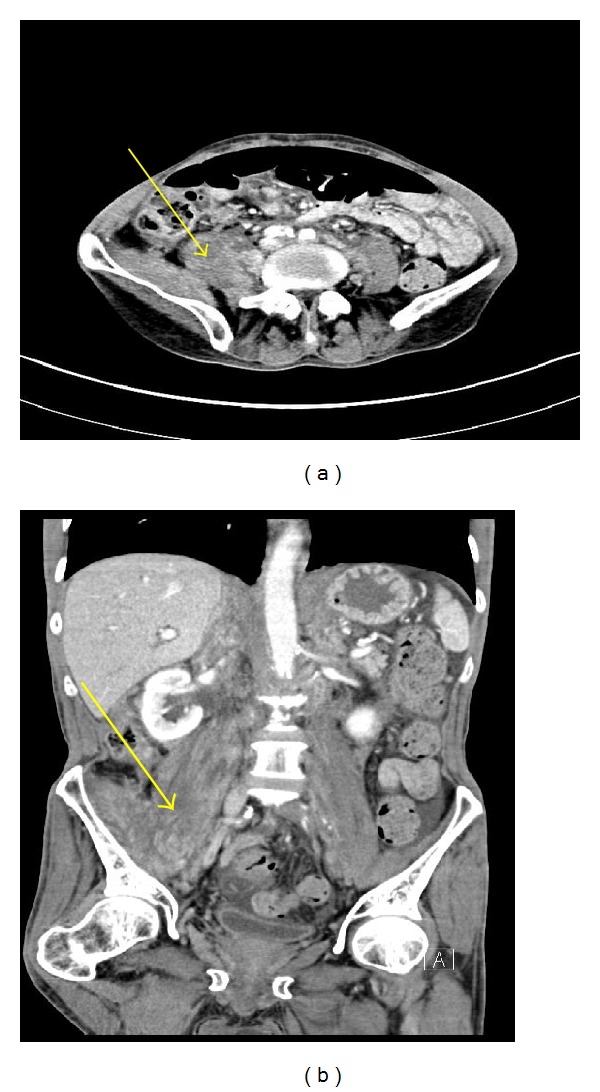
(a) Contrast-enhanced axial CT image through upper pelvis demonstrate enlarged, heterogeneously enhancing right psoas and iliacus muscles with the muscles demonstrating increased and prominent striations (as pointed by arrow). Left psoas is normal. (b) Contrast-enhanced coronal CT image through the abdomen and the pelvis demonstrate enlarged, heterogeneously enhancing right psoas and iliacus muscles (as pointed by arrow) with lateral and anterior displacement of right kidney. No fluid collection is seen.
